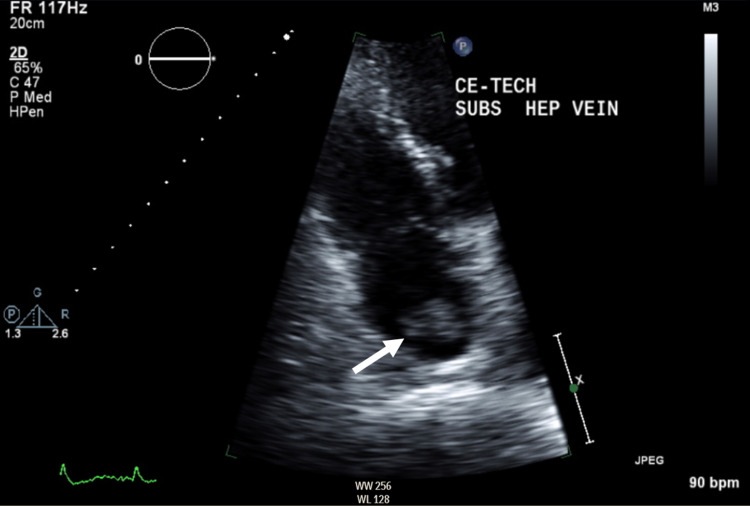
Transthoracic echocardiogram showing the presence of right atrial mass.
